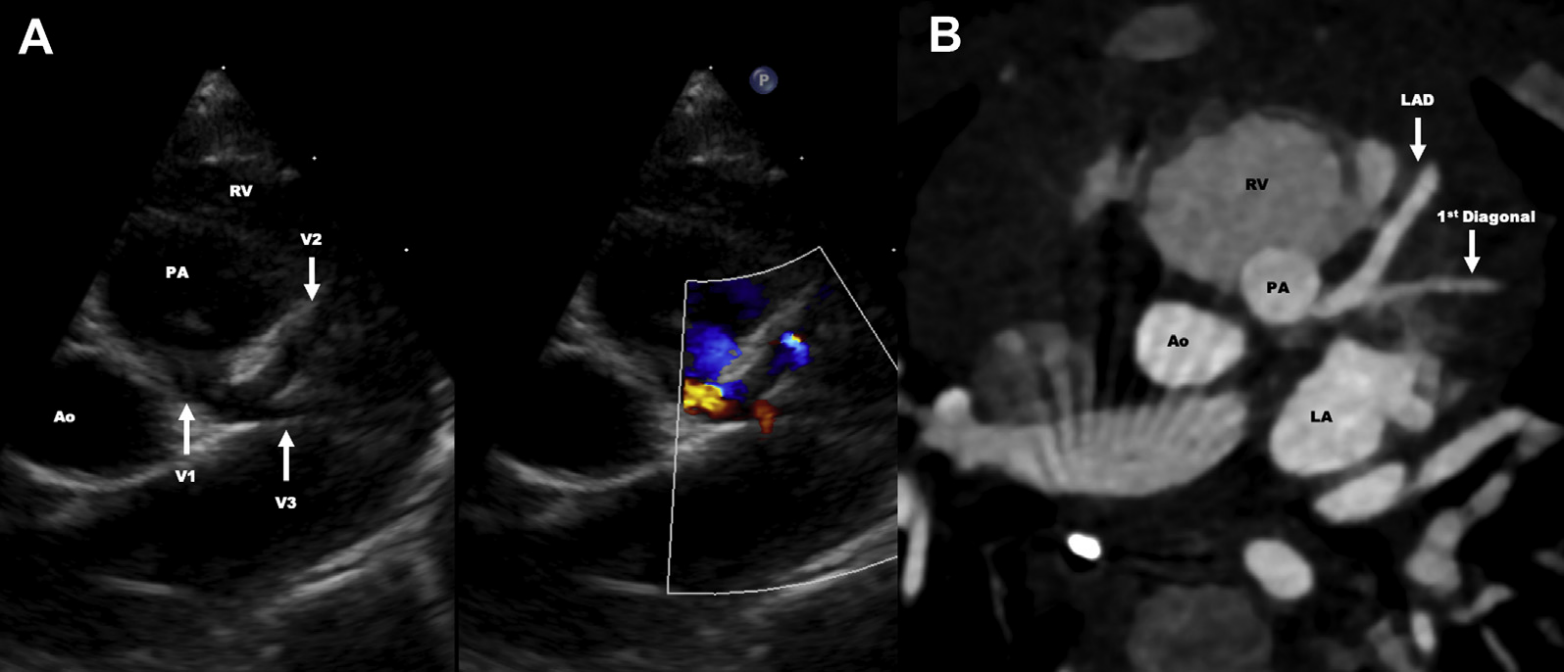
Echocardiography and Computed Tomography Images of the Anomalous Coronary Artery Takeoff From the PA (A) Parasternal short-axis view at the level of the base showing a coronary artery taking off from the pulmonary artery (PA) and bifurcating shortly thereafter. On echocardiography, the bifurcation was presumed to be left coronary artery (V1) branching into the left anterior descending (LAD) (V2) and the left circumflex artery (V3). Reversal of flow can be seen at the takeoff of the coronary artery in the Doppler image. (B) Axial computed tomographic angiography image confirming the origin of the anomalous vessel from the main PA. However, the vessel originating from the PA, and the bifurcation anatomy was confirmed as LAD coronary artery coursing into the anterior interventricular groove and its first diagonal branch supplying the anterolateral wall of the left ventricle. Ao = aorta; LA = left atrium; RV = right ventricle.
